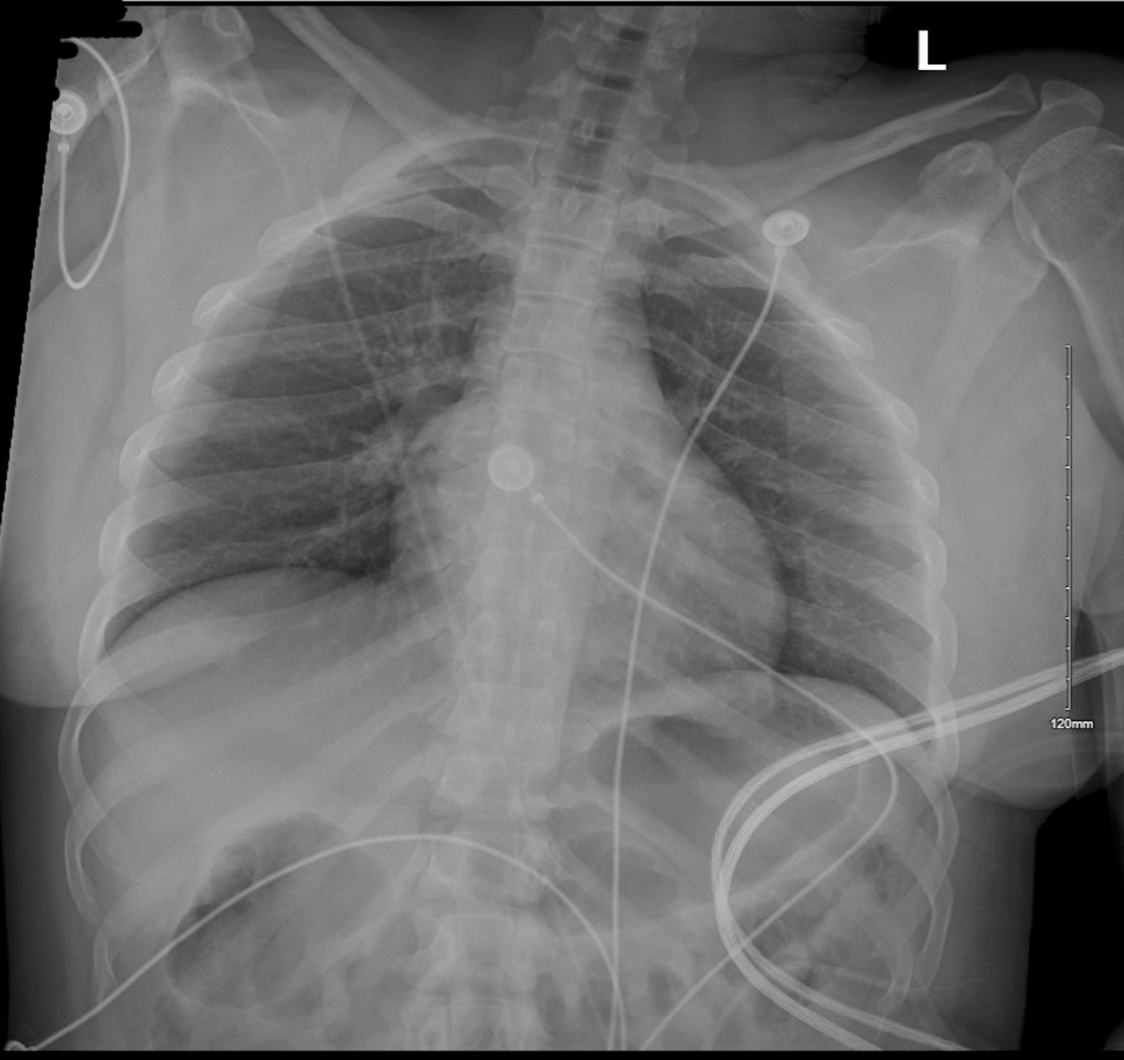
The chest X-ray was normal.
